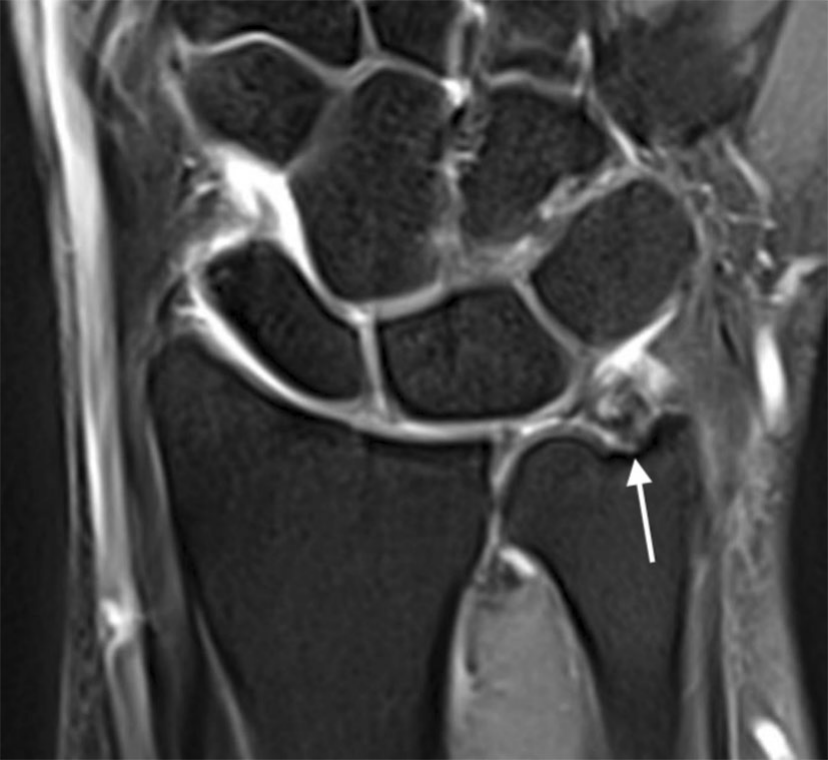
Coronal T2-weighted wrist MRI showing a TFCC tear (arrow)
